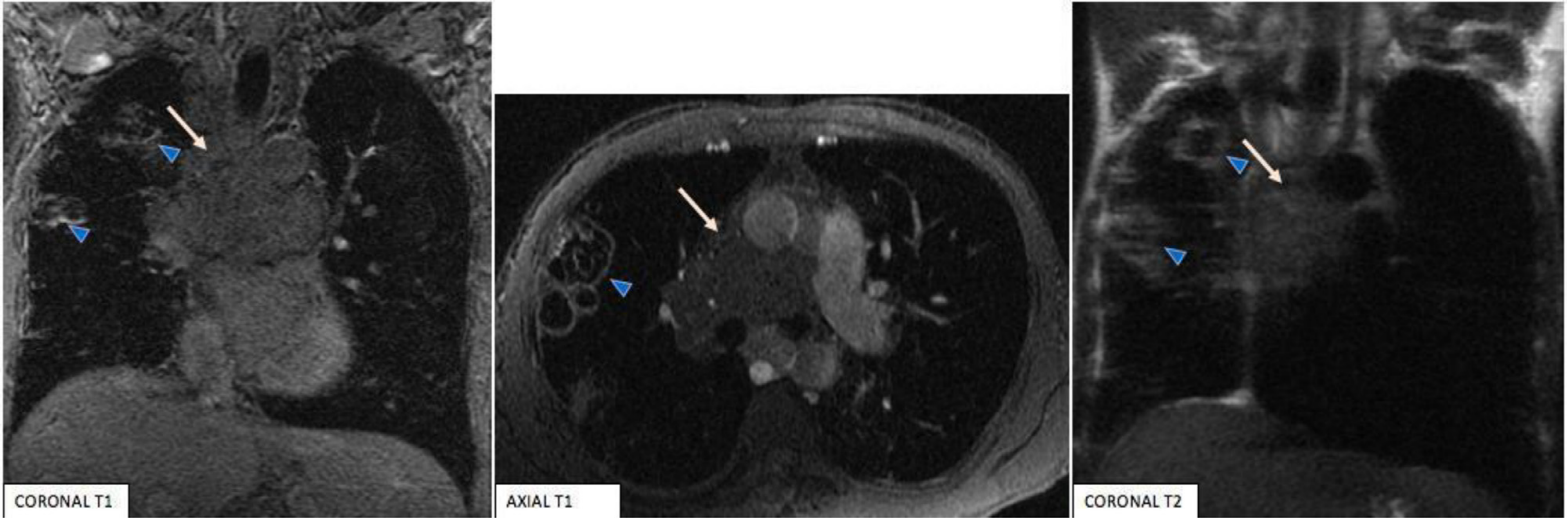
Chest MRI showing ill-defined infiltrative mediastinal fibrous soft tissue (Arrows), presenting low signal on T1 and T2-weighted images. We note also the pulmonary cavitary lesions (arrowheads).
